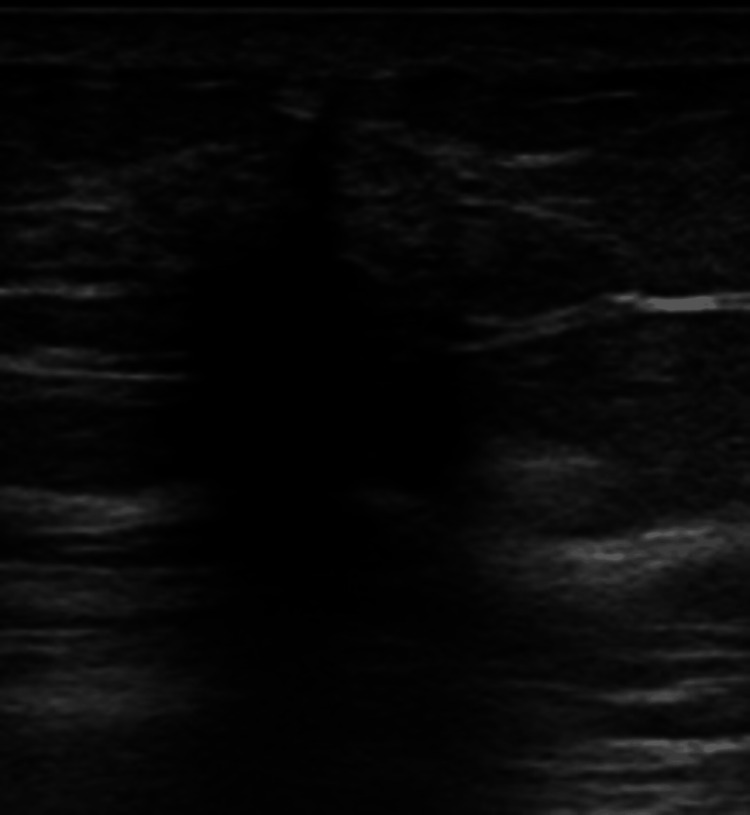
Breast ultrasound showing an irregular hypoechoic mass with indistinct margins and posterior acoustic shadowing.
